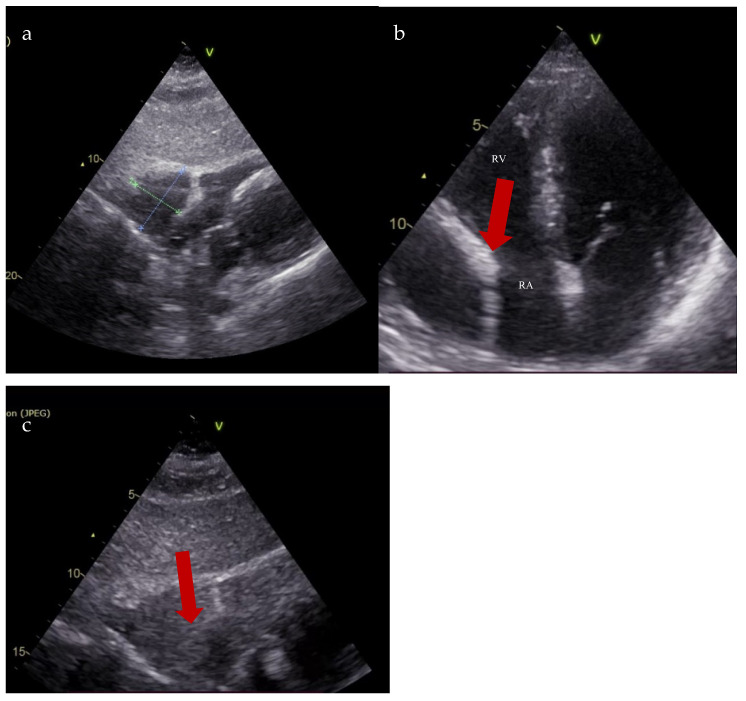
Transthoracic echocardiography. (a). Subcostal view: right atrial diverticulum 36 mm × 49 mm; (b). 4-chamber apical section: right atrial diverticulum with systolic collapse of the right atrium (red arrow); (c). Saline contrast test—subcostal section: communication between right atrial diverticulum and right atrium (red arrow) (RA—right atrium; RV—right ventricle).
